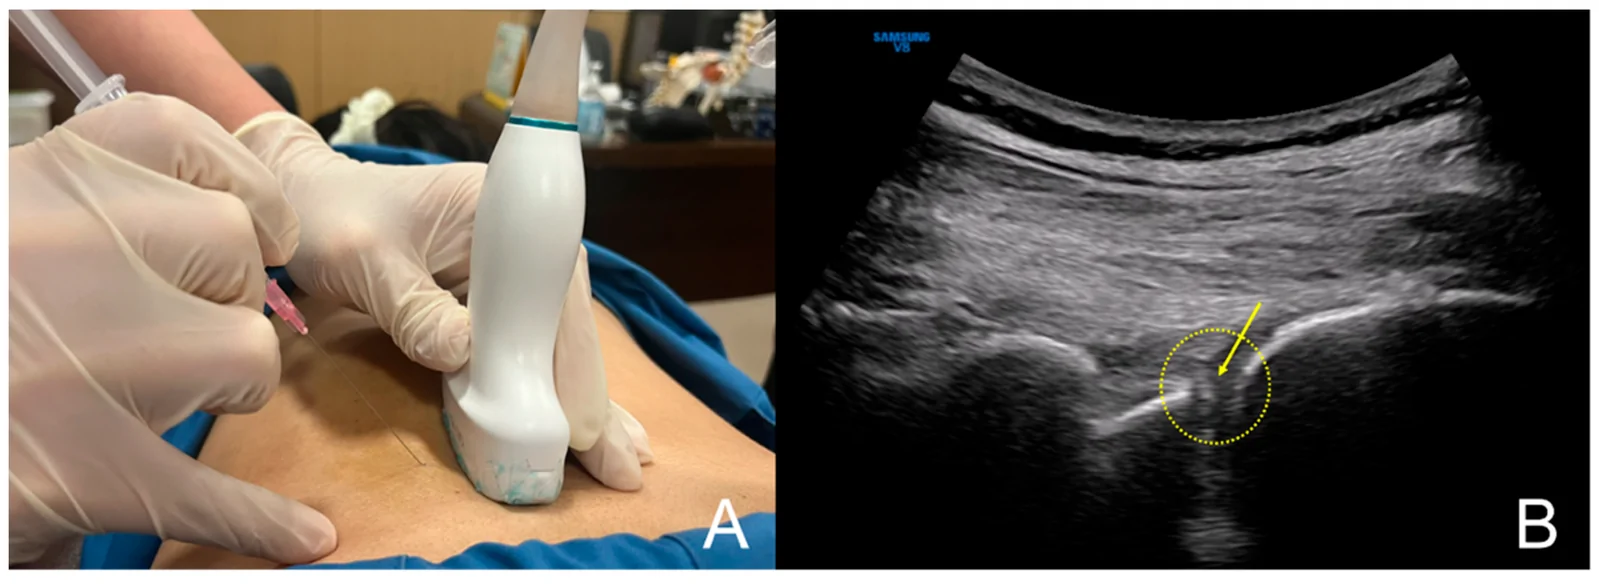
Ultrasound-guided S1 nerve root injection. (A) Out-of-plane approach for ultrasound-guided S1 nerve root pharmacopuncture. (B) The yellow circle indicates the S1 foramen, and the yellow arrow indicates the needle tip injecting the Shinbaro 2 pharmacopuncture solution.
